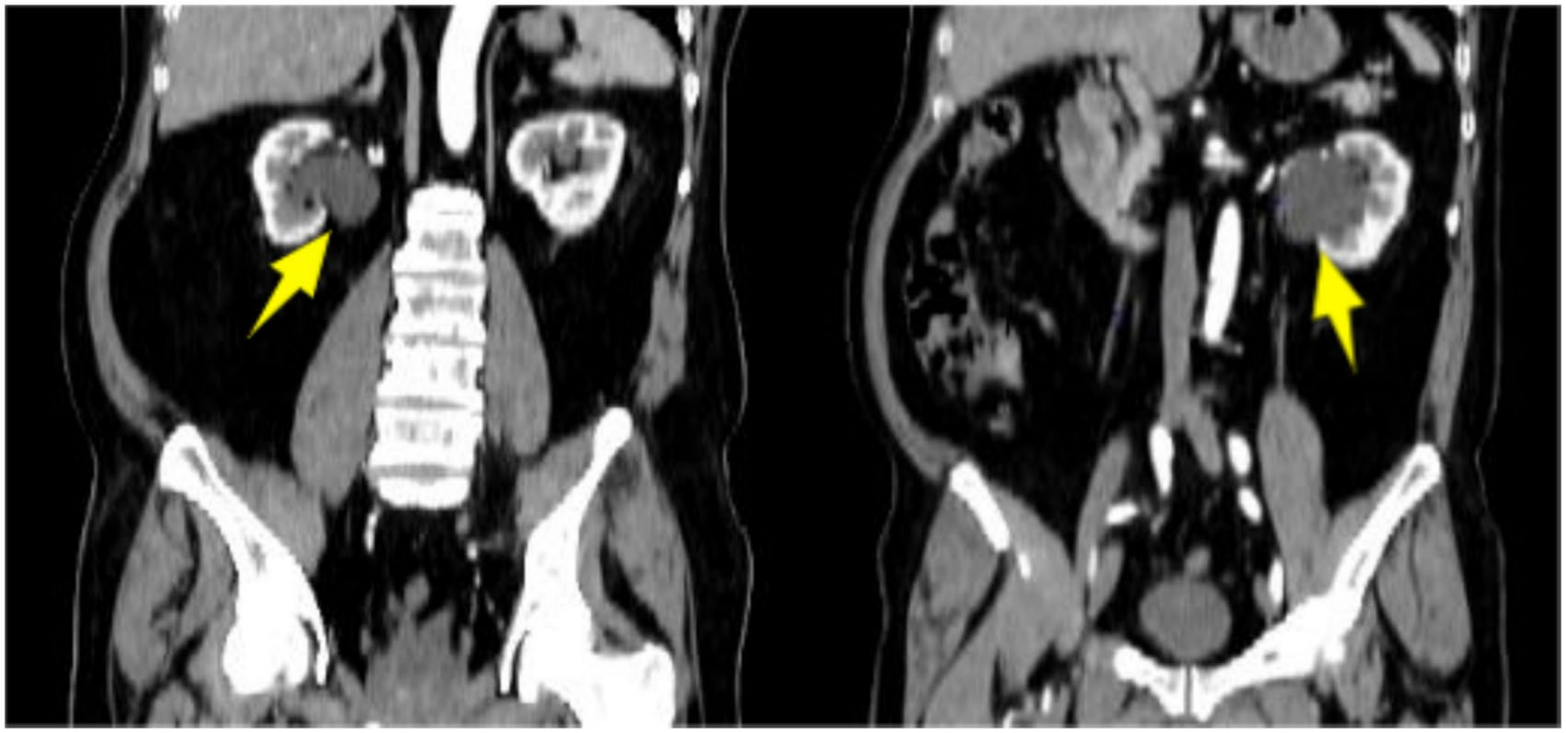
Coronal reformatted contrast-enhanced CT showing cystic low-density lesions in bilateral renal pelves (as indicated by arrows).
